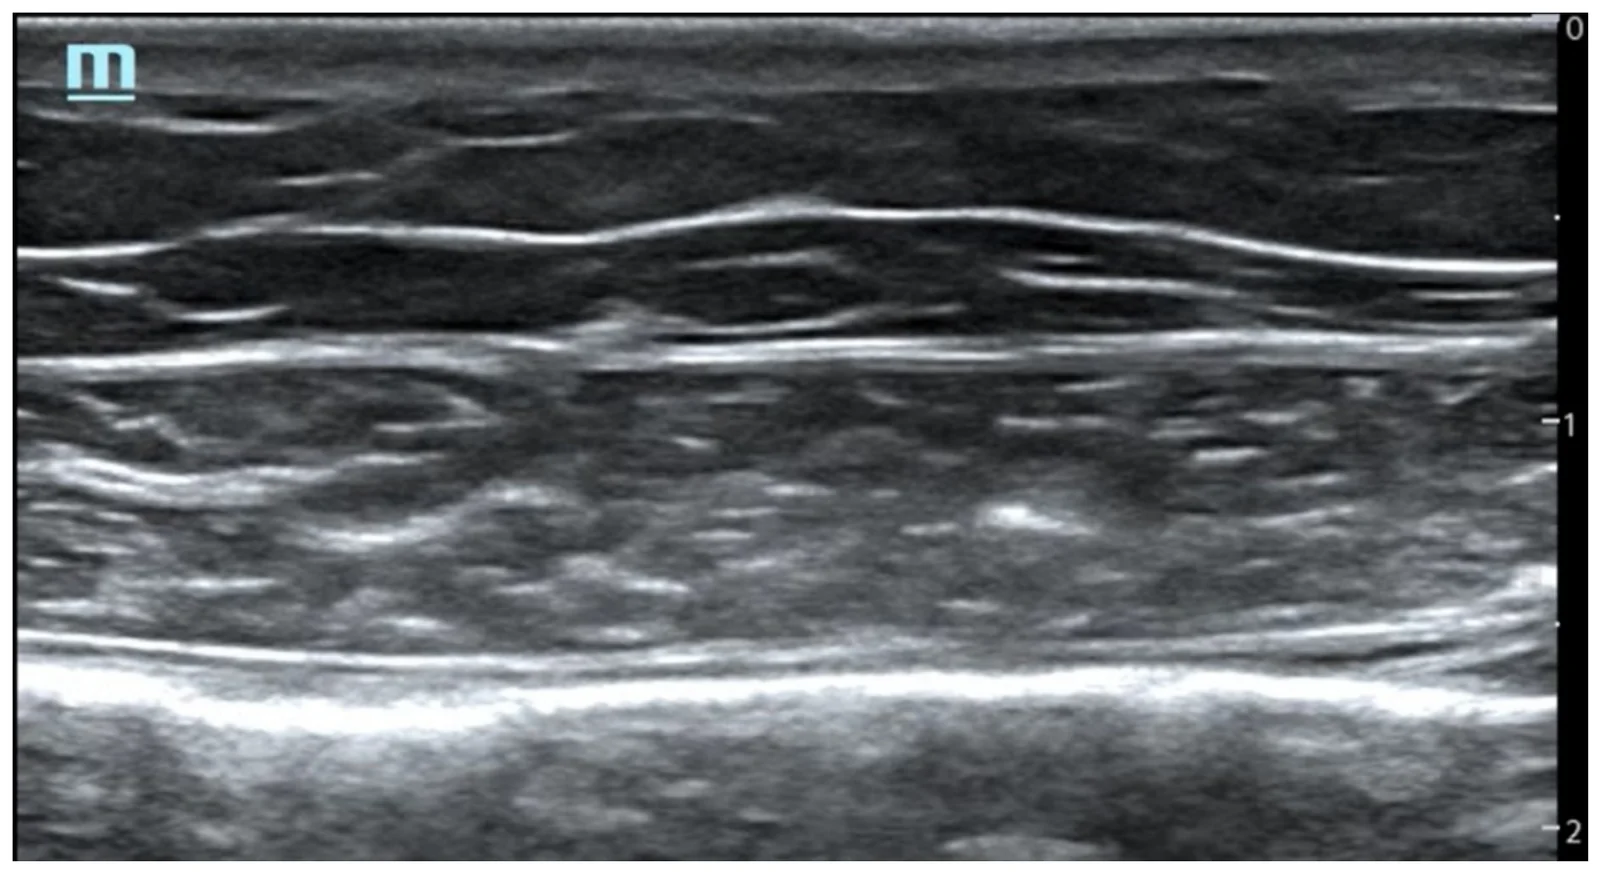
Ultrasound view of rectus abdominis muscle inside its sheath.
